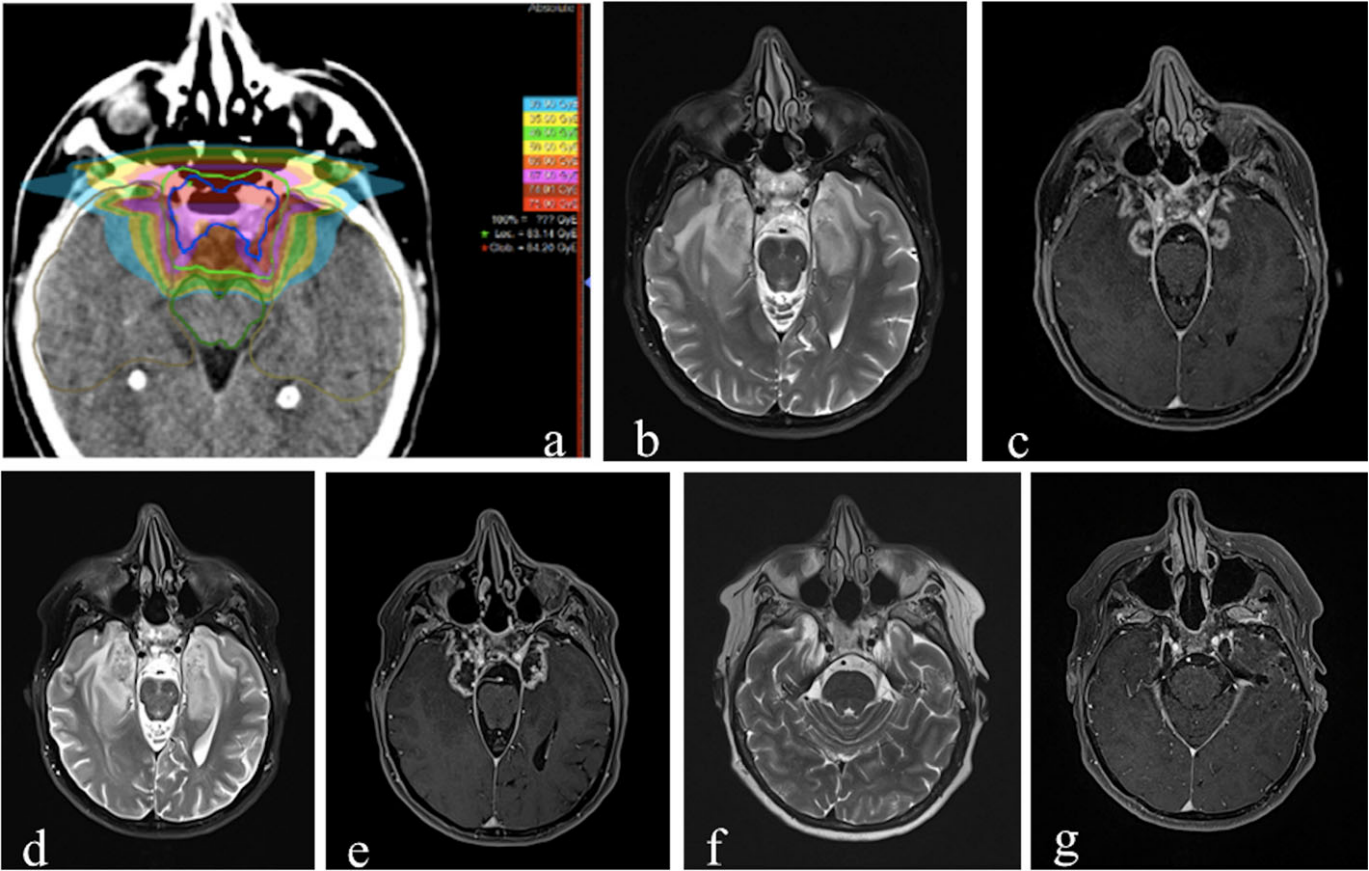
MRI performed in a patient who underwent 74 Gy proton-beam therapy for skull base chordoma. Radiation treatment field (a). After 18 months from the end of the treatment, axial T2-weighted image (b) shows diffuse hyperintensity involving predominantly white matter in temporal lobes and axial postcontrast T1-weighted image (c) demonstrates irregular-shaped enhancing areas in both mesial and polar temporal regions. The morphology of the enhancing lesions shown is in accordance with the radiation treatment field shown in (a). At the following follow-up, performed 21 months after the end of the treatment, axial T2-weighted image (d) and axial postcontrast T1-weighted image (e) show that the lesions and the surrounding edema shown in (b) and (c) have increased in volume. The morphology of the enhancing lesions shown remains in accordance with the radiation treatment field shown in (a). After 48 months from the end of the treatment, T2-weighted image (f) and axial T1 post-contrast-weighted image (g) show the evolution of the alterations, demonstrating focal atrophic changes in both temporal poles according with the evident decrease of extension of the contrast enhancing lesions
